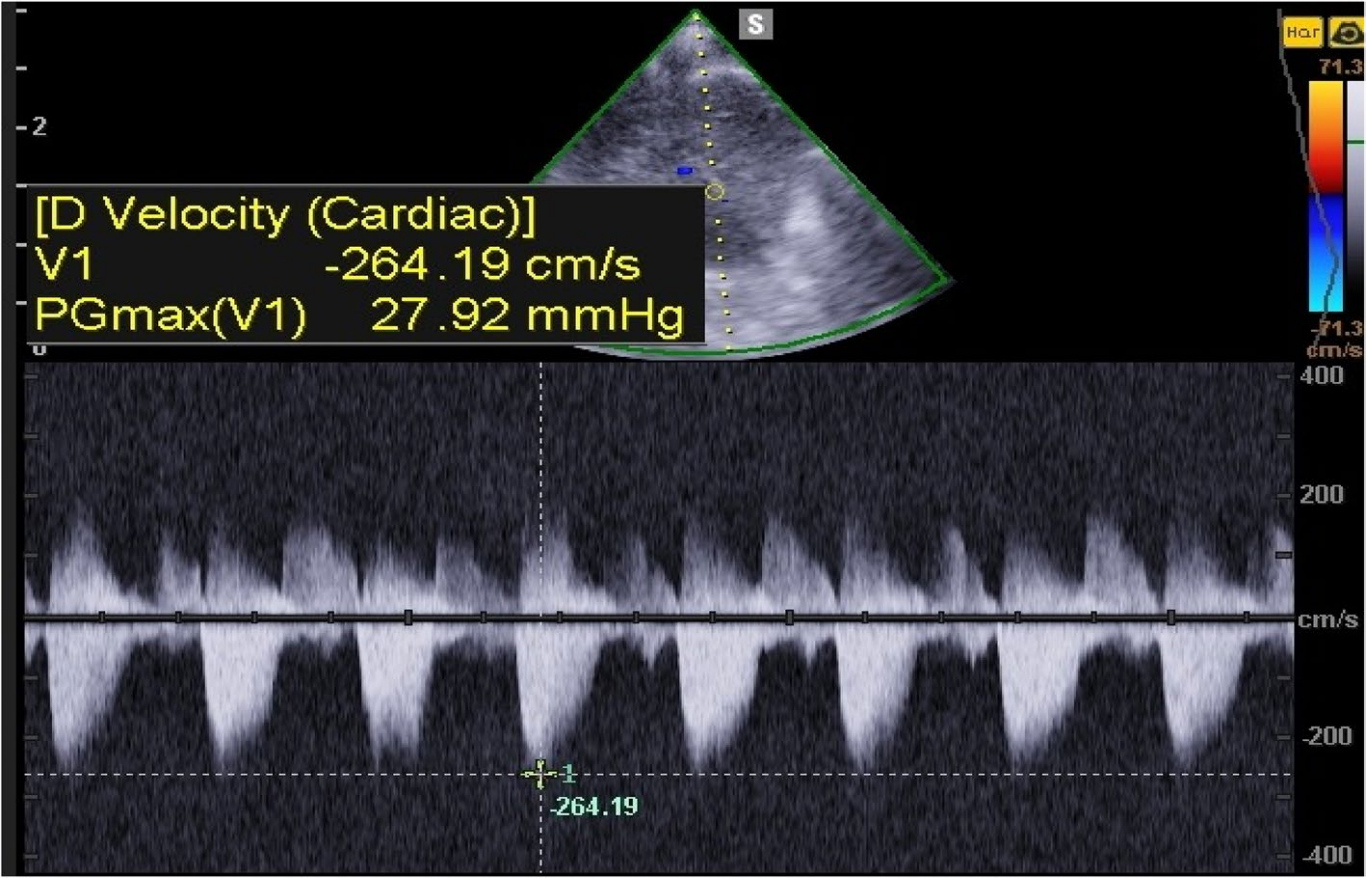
Post procedure transthoracic echocardiography of the patient shows significant reduction in transpulmonary valve gradient, with a mild stenosis
